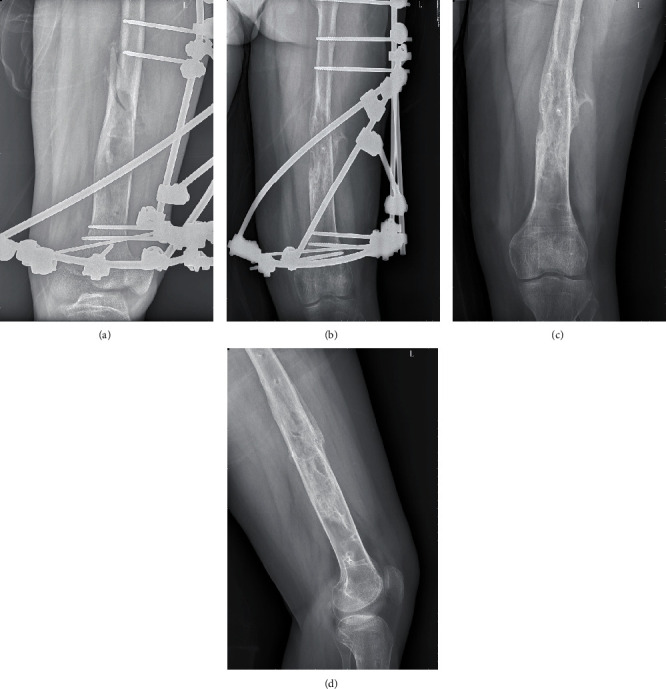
Postoperative x-ray and follow-up. (a) Initial postoperative day, featuring a multiplanar external frame for stabilization. (b) One-week postsurgery, where a secure external fixation is present without any displacement at the fracture site. (c, d) A 2-year follow-up period after surgery, indicating no recurrence.
